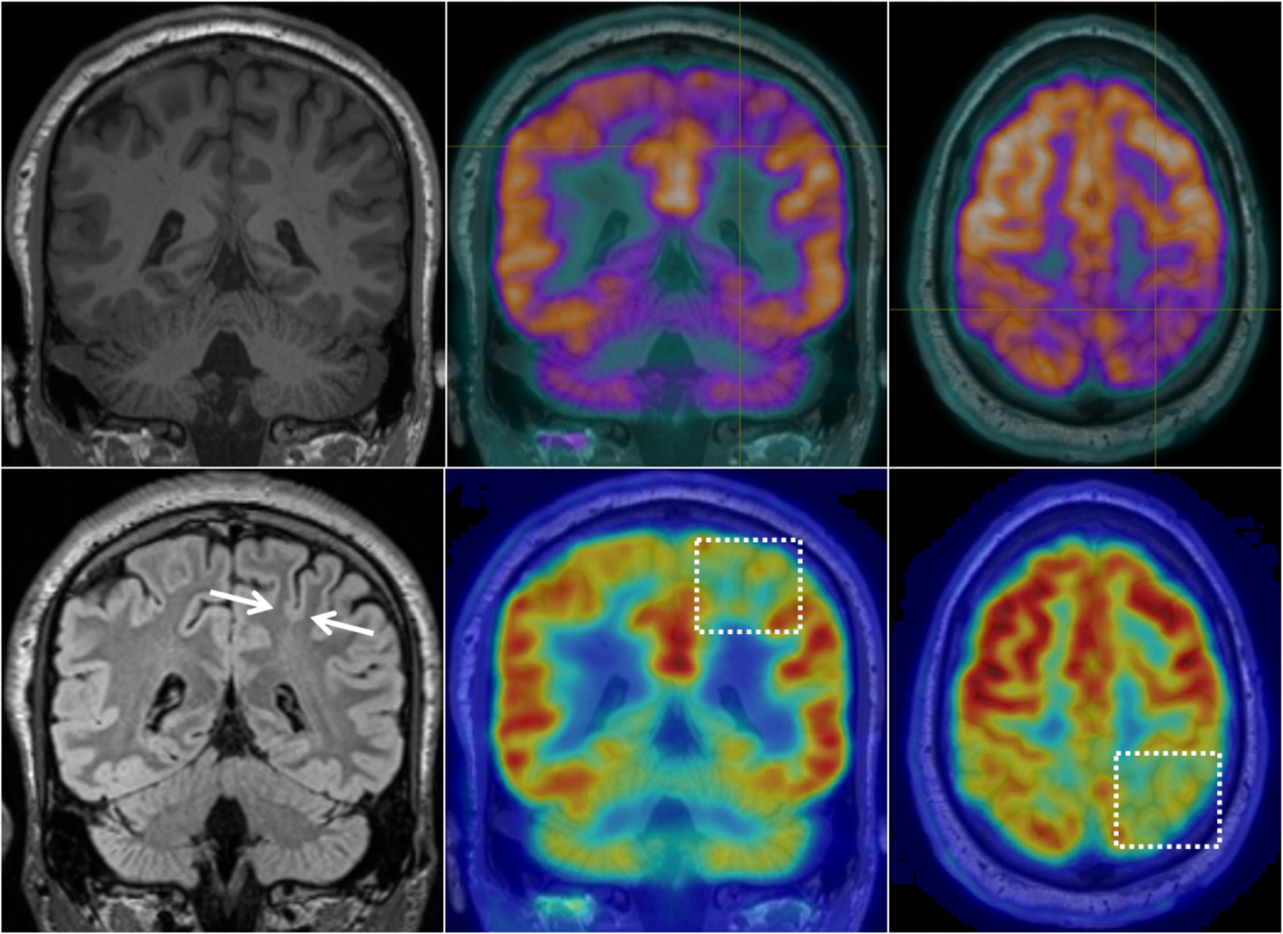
MRI and PET images for an individual with regional PET hypometabolism. Regional hypometabolism was evident in this patient, with gradual decline in activity evident within the boxed area when compared to adjacent cortex. MRI was initially negative, and a subtle BOSD was detected only upon the second review of MRI with co-registered PET in this patient (arrows shown on FLAIR in bottom left image). This patient was seizure free following focal resection of the BOSD
